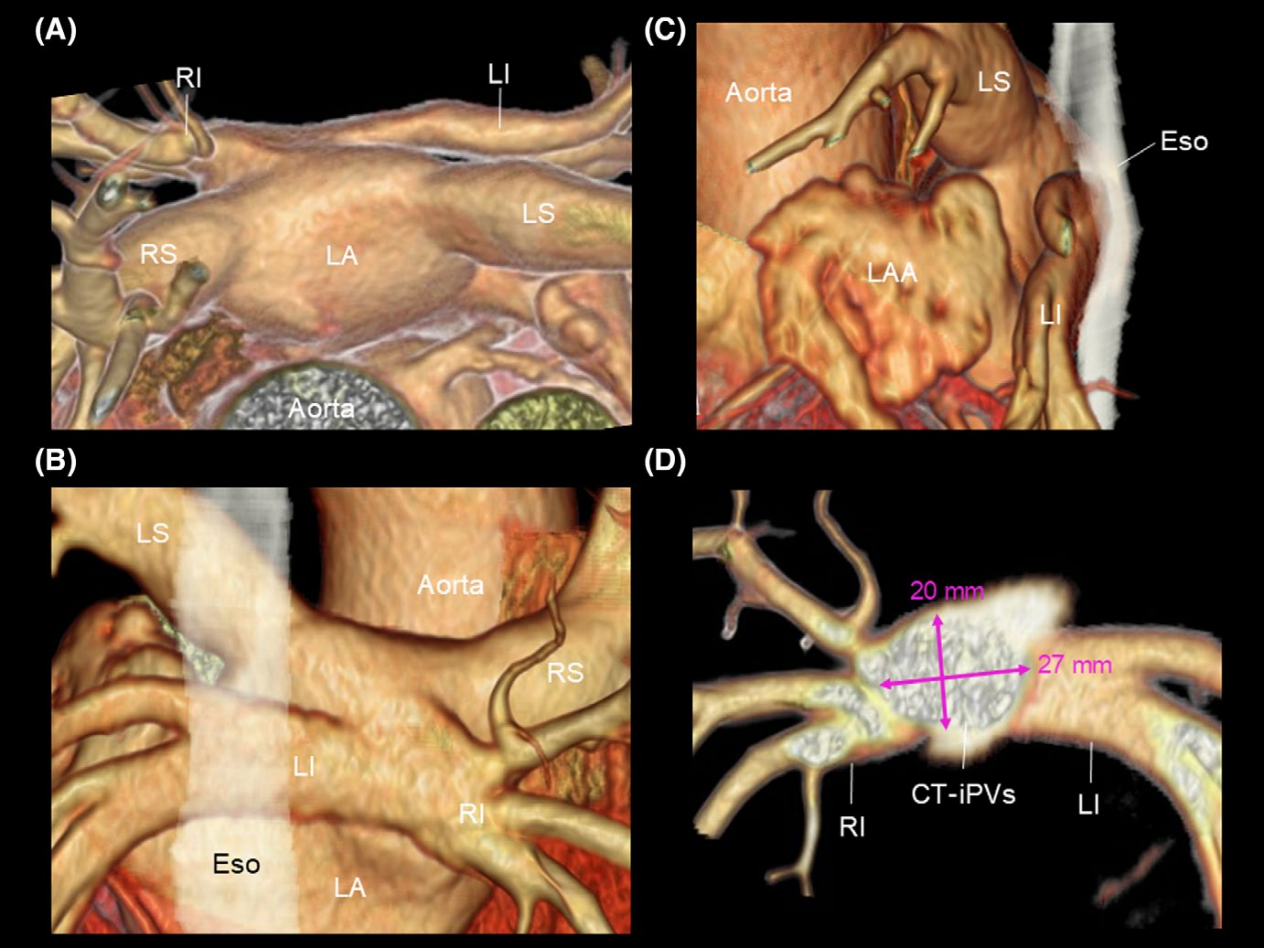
Three‐dimensional computed tomography reconstruction images before ablation. (A) Superior view, (B) posterior view, (C) left lateral view, (D) a cross‐section of the CT‐iPVs in the anterior view. The esophagus is indicated by a white translucent zone. CT‐iPVs, common trunk of inferior pulmonary veins; Eso, esophagus; LA, left atrium; LI (S), left inferior (superior) pulmonary vein; RI (S), right inferior (superior) pulmonary vein.
